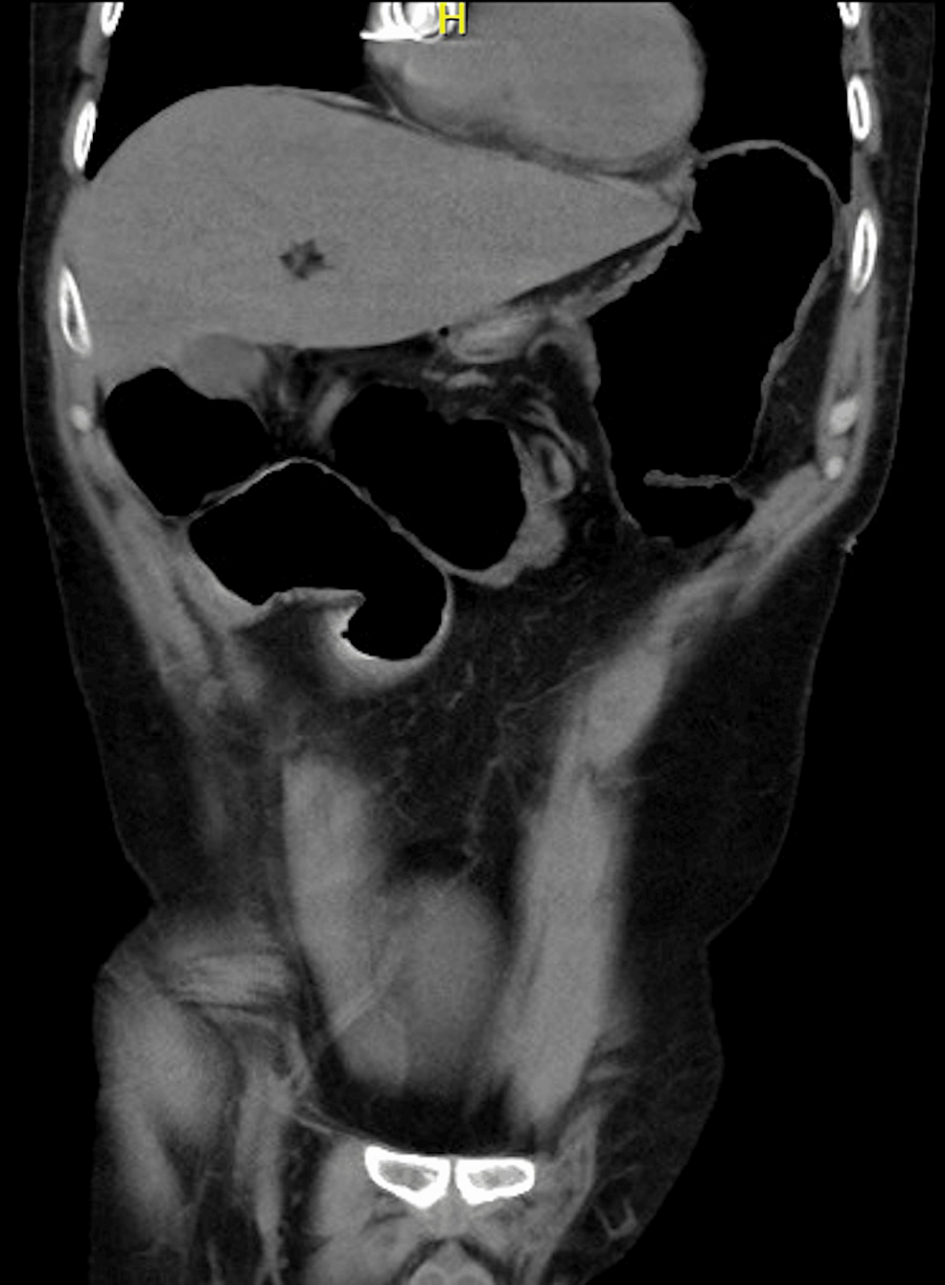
This coronal section of the abdominal CT scan shows extensive dilatation of slender loops from the duodenum.
